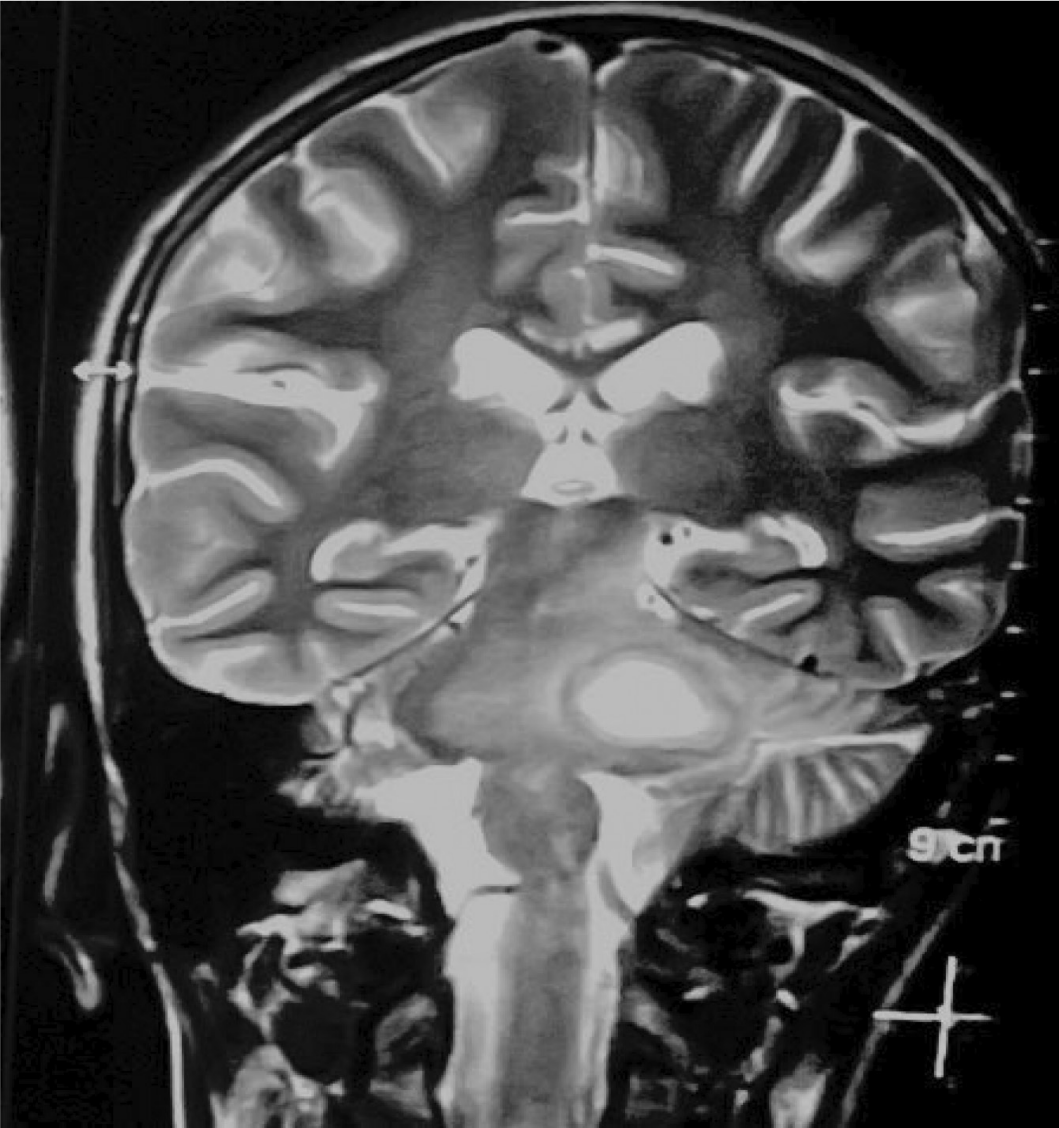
Coronal view of contrast‐enhanced T2 weighted MRI of head showing rim‐enhancing cystic lesion in left pons and within the left side of cerebellopontine angle.
